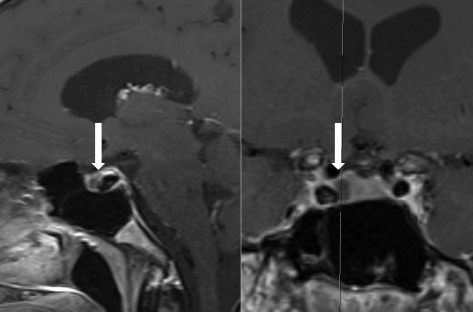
MRI of the pituitary gland. T1 contrast-enhanced images. *Left* image: sagittal view. *Right* image: coronal view. *White* arrow indicates cystic structure in the pituitary
